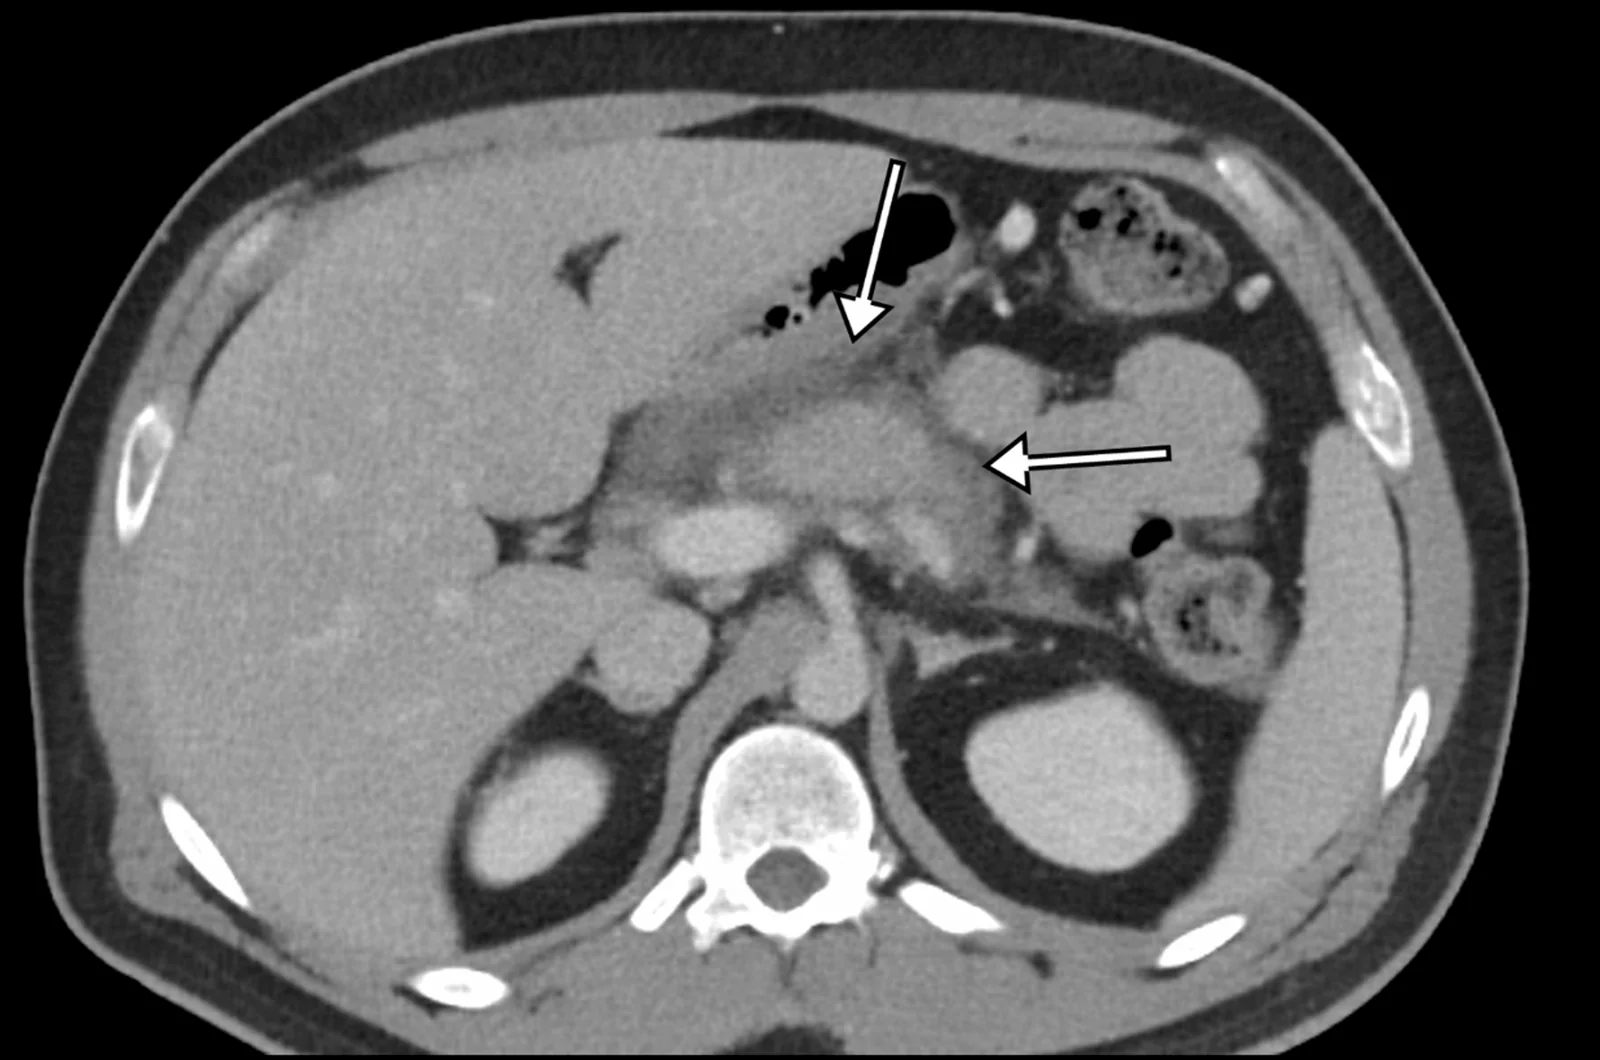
Axial contrast-enhanced computed tomography at the level of the pancreas. Arrows indicate pancreatic fluid tracking along the anterior and left margin of the bulky, ill-defined pancreas, with surrounding peripancreatic fat stranding.
